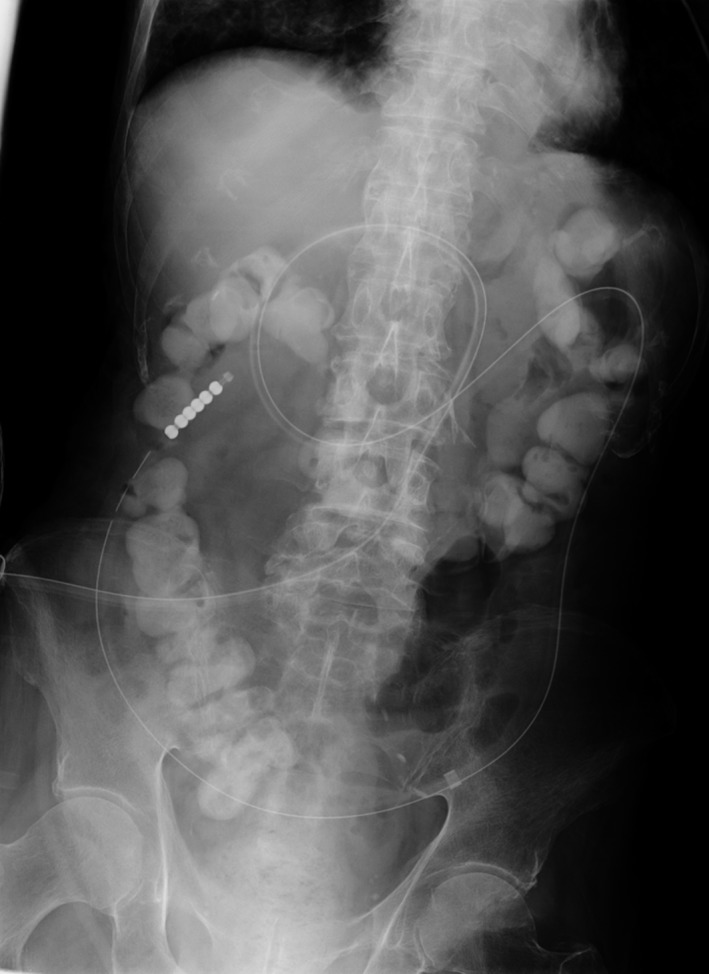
Successful decompression confirmed by abdominal radiograph on day 4.
